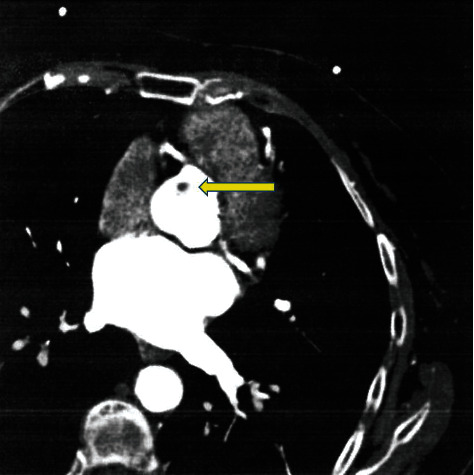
Computed tomography of the heart: the yellow arrow indicates the small round mass located on the right coronary cusp, 11 mm in diameter. Note the origin of the right and left coronary arteries.
